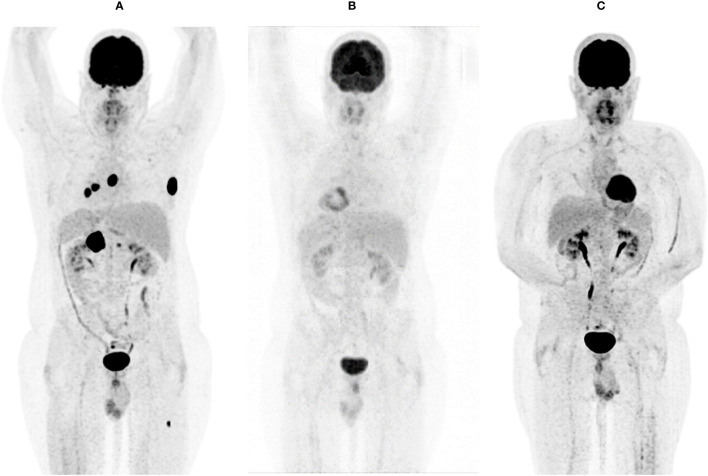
FDG-PET-CT showing systemic metastasis before (A), after three months (B), and after one year (C) of double check point inhibition with ipilimumab and nivolumab.
